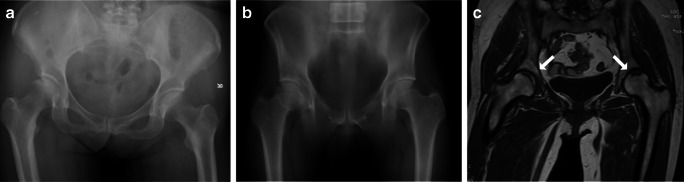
a DR shows no apparent abnormality. b DTS shows (c) CT/MR diagnosis of osteonecrosis of the femoral head
